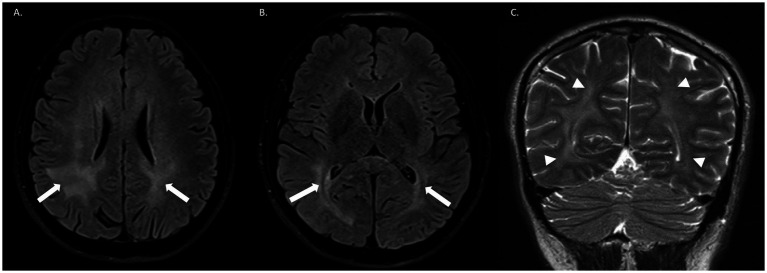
Brain MRI. (A,B) Axial FLAIR sequences show bilateral asymmetric involvement of the white matter, predominantly in the occipital regions, particularly periventricular, without cortical involvement or edema (white arrows). (C) Coronal T2 sequence demonstrates asymmetric leukoencephalopathy with extensive bilateral subcortical white matter damage, more pronounced in the right hemisphere (white arrowheads).
